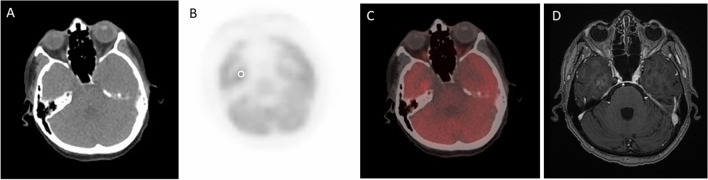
^18^F-FDG PET/CT and contrast-enhanced MRI images of a HER2-negative breast cancer metastasis. Non-contrast CT (A), ^18^F-FDG PET (B), fused ^18^F-FDG PET and CT (C), and axial T1-weighted post-contrast (D) images of a HER2-negative breast cancer brain metastasis in a 68-year-old woman. A representative region of interest is shown in white. This lesion had an SUVmax ratio of 2.19. ^18^F-FDG = ^18^F-fluorodeoxyglucose. SUVmax = maximum standardized uptake value
